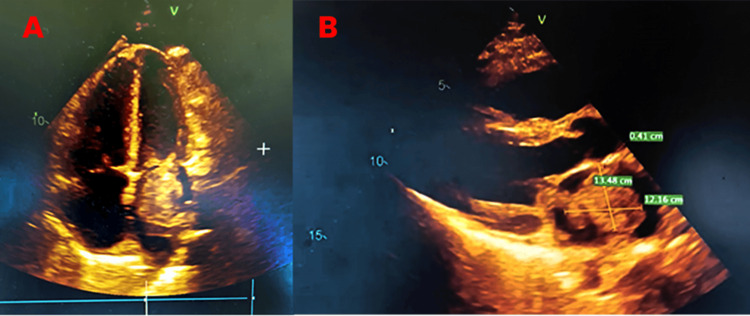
(A) Transthoracic echocardiography showing left atrial myxoma prolapsing into the mitral valve; (B) measurement of left atrial myxoma and mitral valve obstruction on echocardiography
